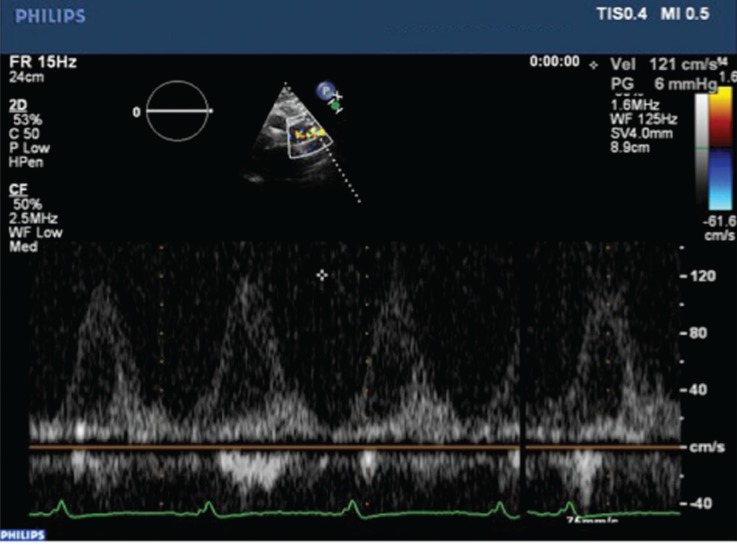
Outflow cannula velocity measurement with Doppler.
